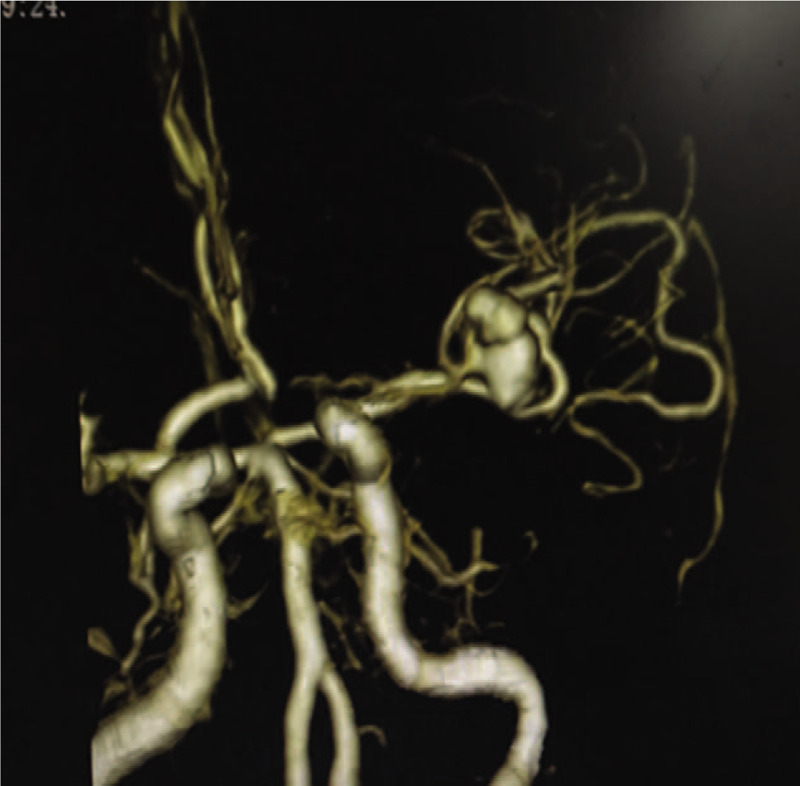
Computed tomography angiography scan showing aneurysm found in the left middle cerebral artery.
